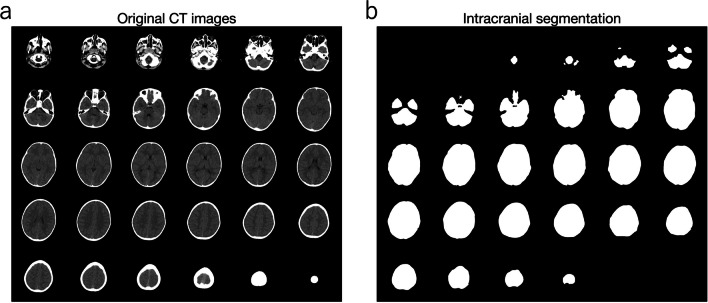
Representative segmentation. Head CT images acquired from a four-year-old male are presented (a), and segmentations focusing on the intracranial region are shown (b)
